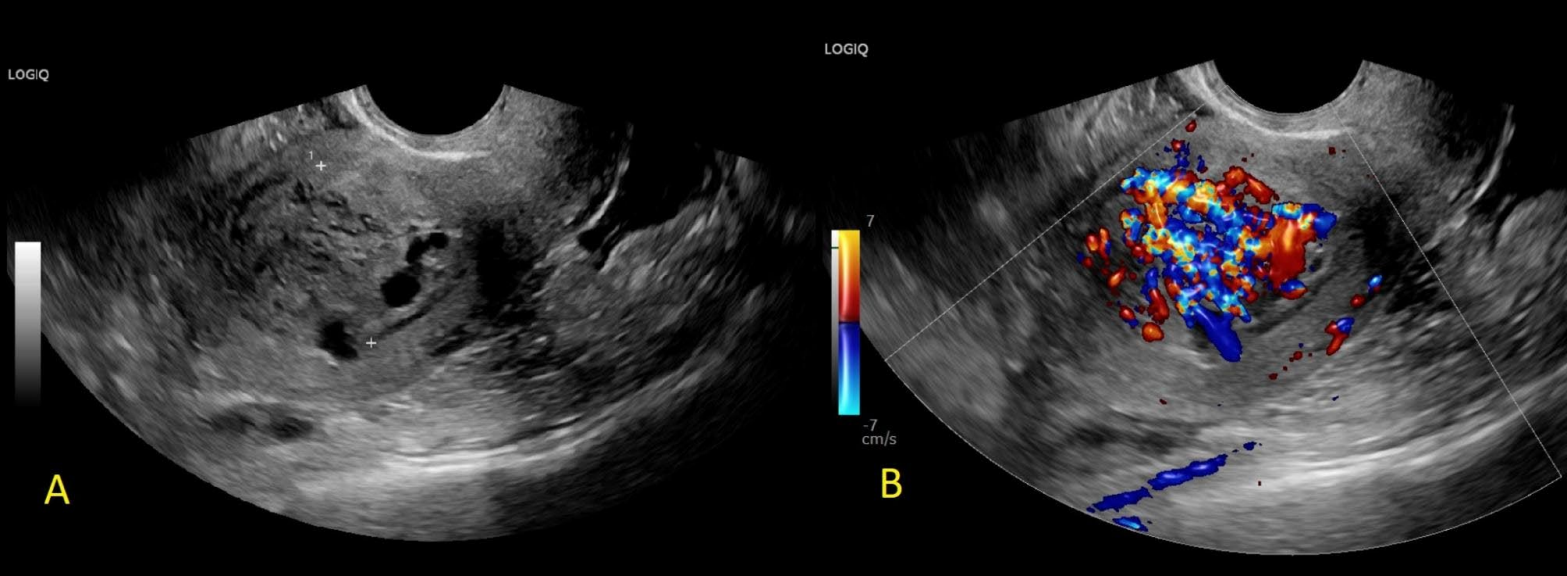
Transvaginal sagittal 2D ultrasound (A) with color flow doppler (B) demonstrating a new mass in the endometrial cavity on presentation to the outside emergency department eight weeks after medication abortion. Comment from radiology was, “Markedly thickened endometrium with heterogeneous appearance and marked vascularity.”
